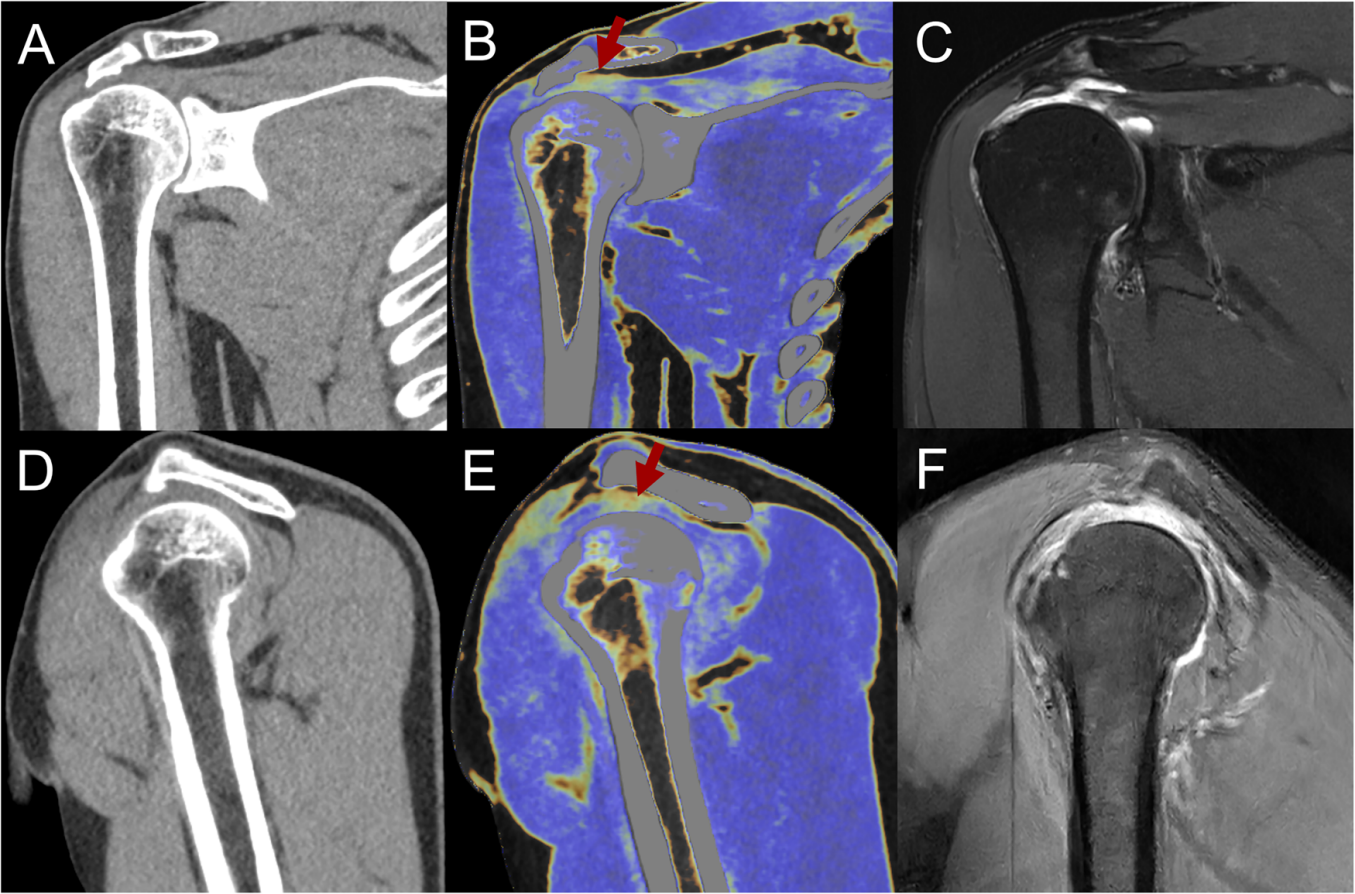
Images for a 73-year-old man experiencing chronic right shoulder pain with limited motion and a positive Neer impingement test. A, D At standard CT, acromion osteomalacia and subacromial gap narrowing. B, E After MMD reconstruction, images detected acromion impingement with supraspinatus, infraspinatus, and subscapularis tendon tears (red arrows). C, F Noncontrast MRI oblique coronal and sagittal views show fat-suppressed proton density-weighted images of superior acromial impingement with the rotator cuff full-thickness tear. MMD, multi-material decomposition
